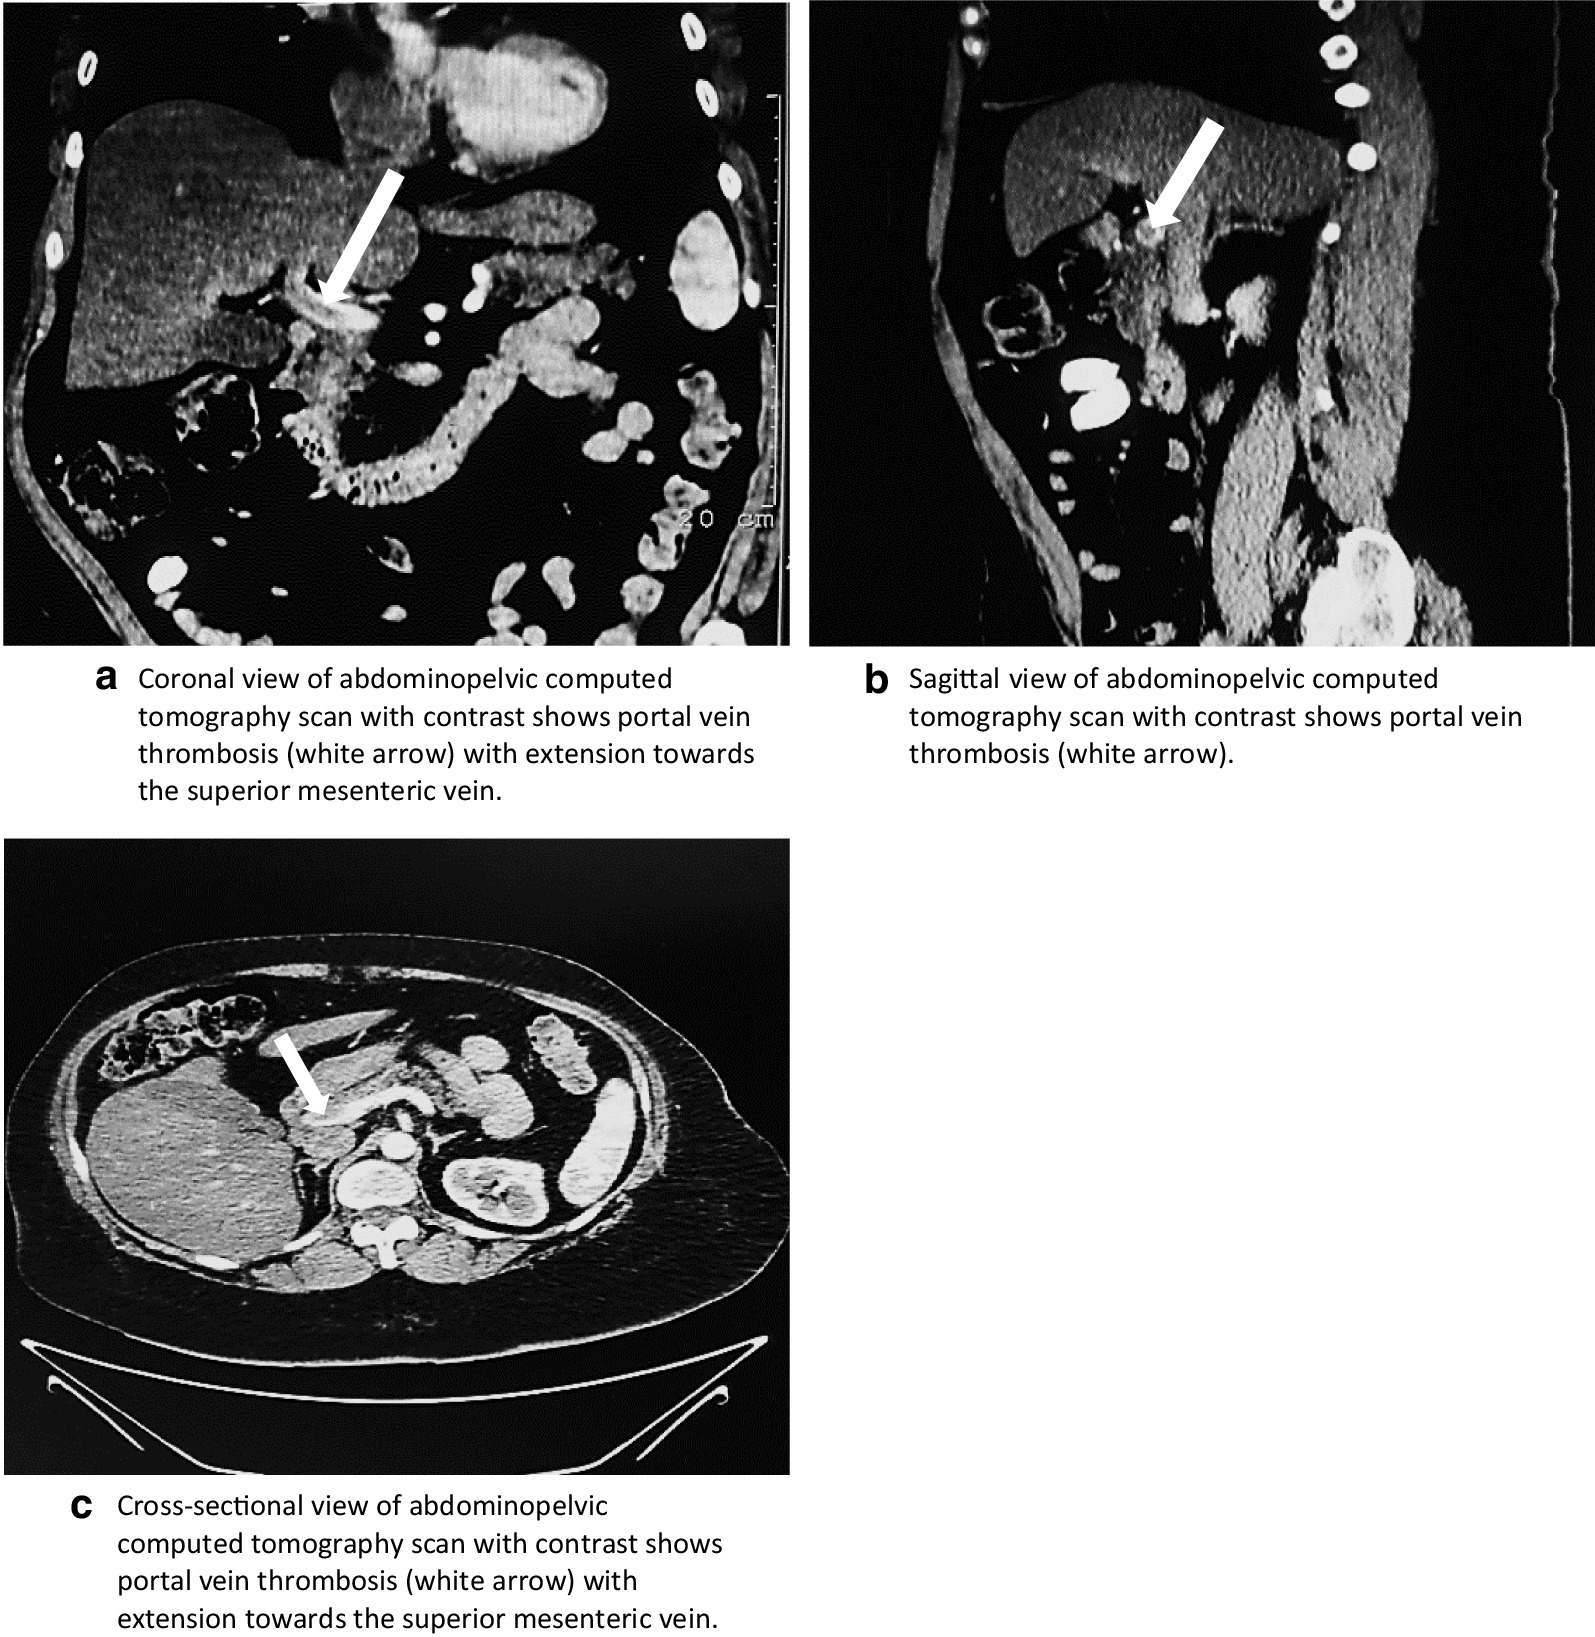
Coronal (a), sagittal (b) and cross-sectional (c) views of abdominopelvic computed tomography scan with contrast demonstrating portal vein thrombosis (white arrows) with extension to the superior mesenteric vein
